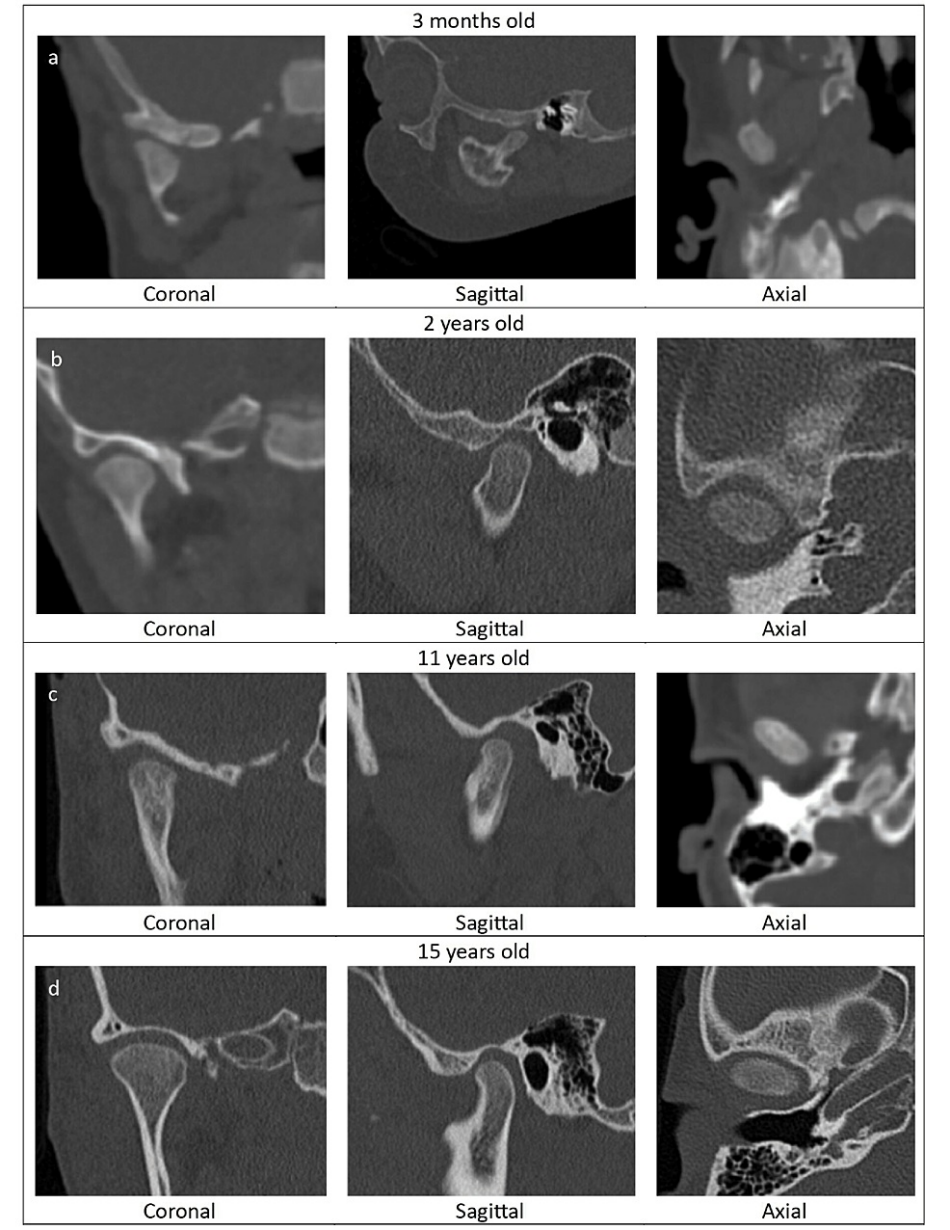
Computed tomography of the temporomandibular joint through childhood (a) CT head without contrast showing TMJ in the coronal (left), sagittal (middle), and axial planes (right) at three months old. The glenoid fossa is flat. (b) CT head without contrast showing TMJ in the coronal (left), sagittal (middle), and axial planes (right) at two years old. (c) CT head without contrast showing TMJ in the coronal (left), sagittal (middle), and axial planes (right) at 11 years old. (d) CT head without contrast showing TMJ in the coronal (left), sagittal (middle), and axial planes (right) at 15 years old. The glenoid fossa is concave making the joint more stable. CT: computed tomography; TMJ: temporomandibular joint
